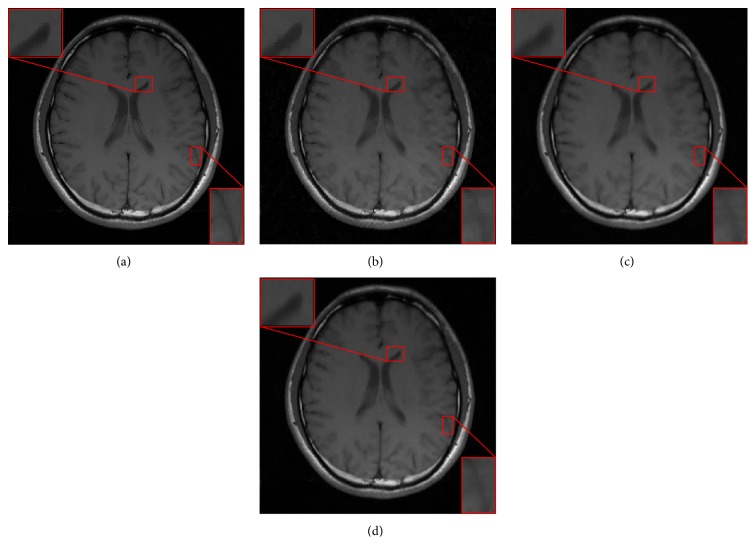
(a) Reference. Reconstructed images from the radial sampling mask with accelerating factor *R* = 4 by (b) CSMRI-TV, (c) DLMRI, and (d) our proposed DDTF-MRI, respectively.
